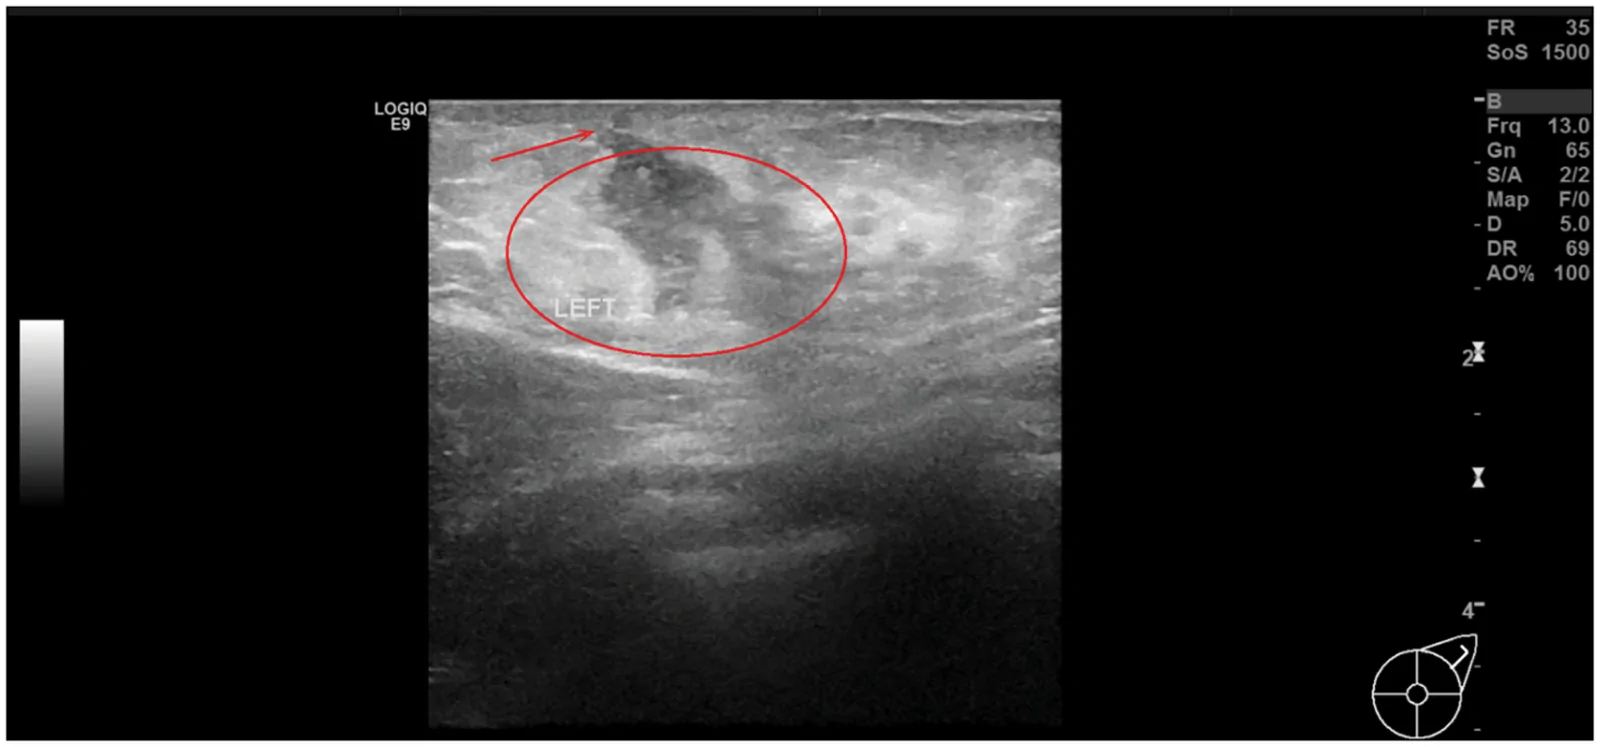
Ultrasound of left axillary accessory breast tissue at initial presentation: A breast-like structure contains patchy hypoechoic areas (red ellipse), some extending superficially toward the skin surface (red arrow).
